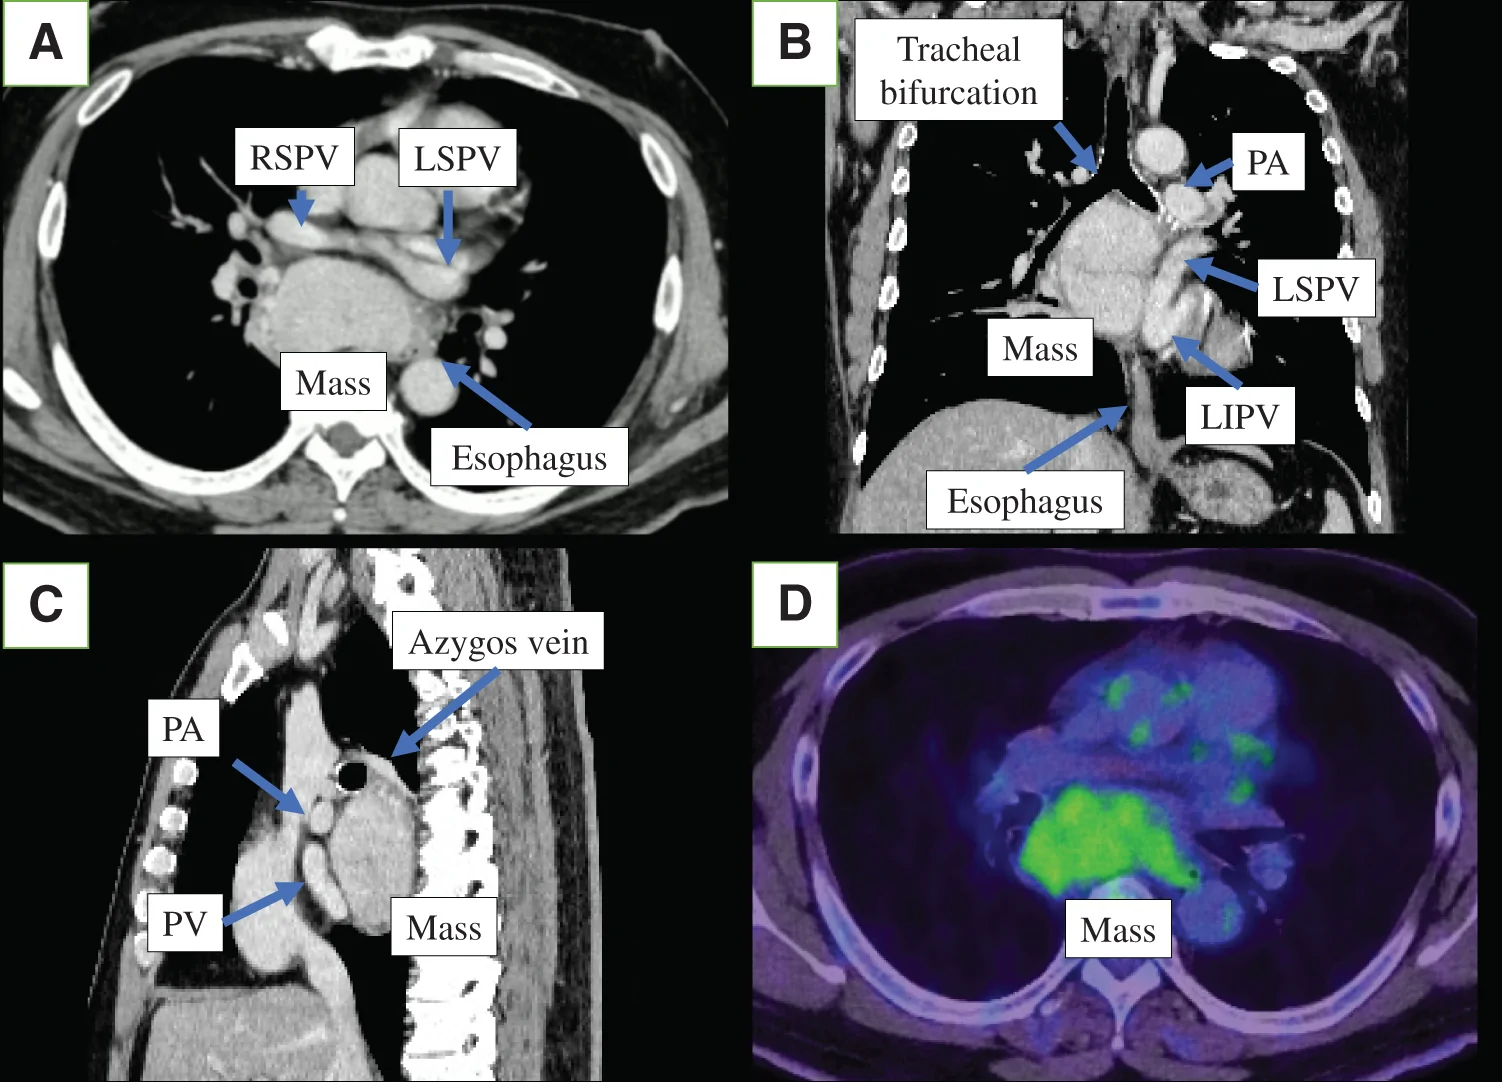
Preoperative imaging findings. (A) Axial contrast-enhanced CT showing a 9.2 × 6.4 cm middle mediastinal mass beneath the tracheal bifurcation. (B) Coronal contrast-enhanced CT showing the relationship between the tumor and adjacent structures, including the tracheal bifurcation and azygos vein. (C) Sagittal contrast-enhanced CT showing the subcarinal location of the tumor and its proximity to the esophagus and major mediastinal structures. (D) PET/CT showing mild uptake, with a maximum standardized uptake value of 3.9. LIPV, left inferior pulmonary vein; LSPV, left superior pulmonary vein; PA, pulmonary artery; PV, pulmonary vein; RSPV, right superior pulmonary vein; SPV, superior pulmonary vein
